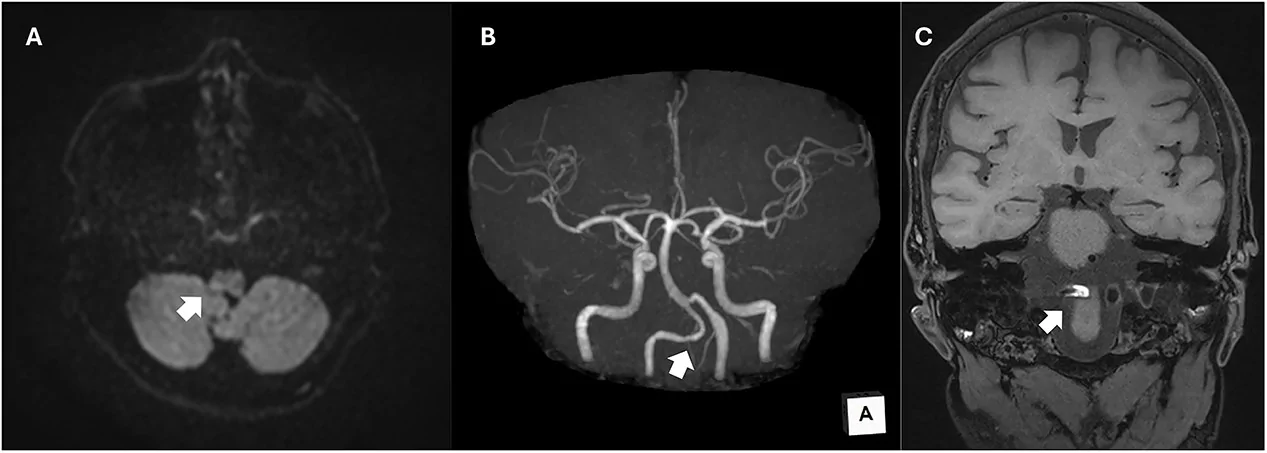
Representative case of IAD involving a patient with medullary infarction. (A) on day 0 (day of admission), DWI demonstrated an acute infarction in the right lateral medulla oblongata (white arrow, A). (B) on day 0, 3D time-of-flight MRA showed focal stenosis of the affected right vertebral artery, without definitive evidence of dissection (white arrow, B). (C) on day 8, VW-MRI demonstrated an intramural haematoma in the right intracranial vertebral artery (white arrow, C). Abbreviations: DWI = diffusion-weighted imaging; IAD = intracranial artery dissection; MRA = magnetic resonance angiography; VW-MRI = vessel wall MRI.
